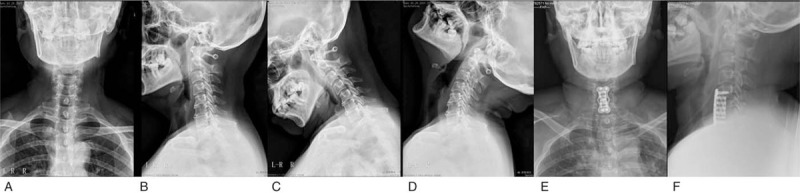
Radiographs of the cervical spine showed decreased cervical curvature, hyperosteogenesis at the edge of the C5, C6 and C7, and narrowing intervertebral space of the C5-C6 and C6-7. A, B represents the anteroposterior and lateral position respectively. C, D indicates the dynamic position. E, F shows a good position of the internal fixation.
